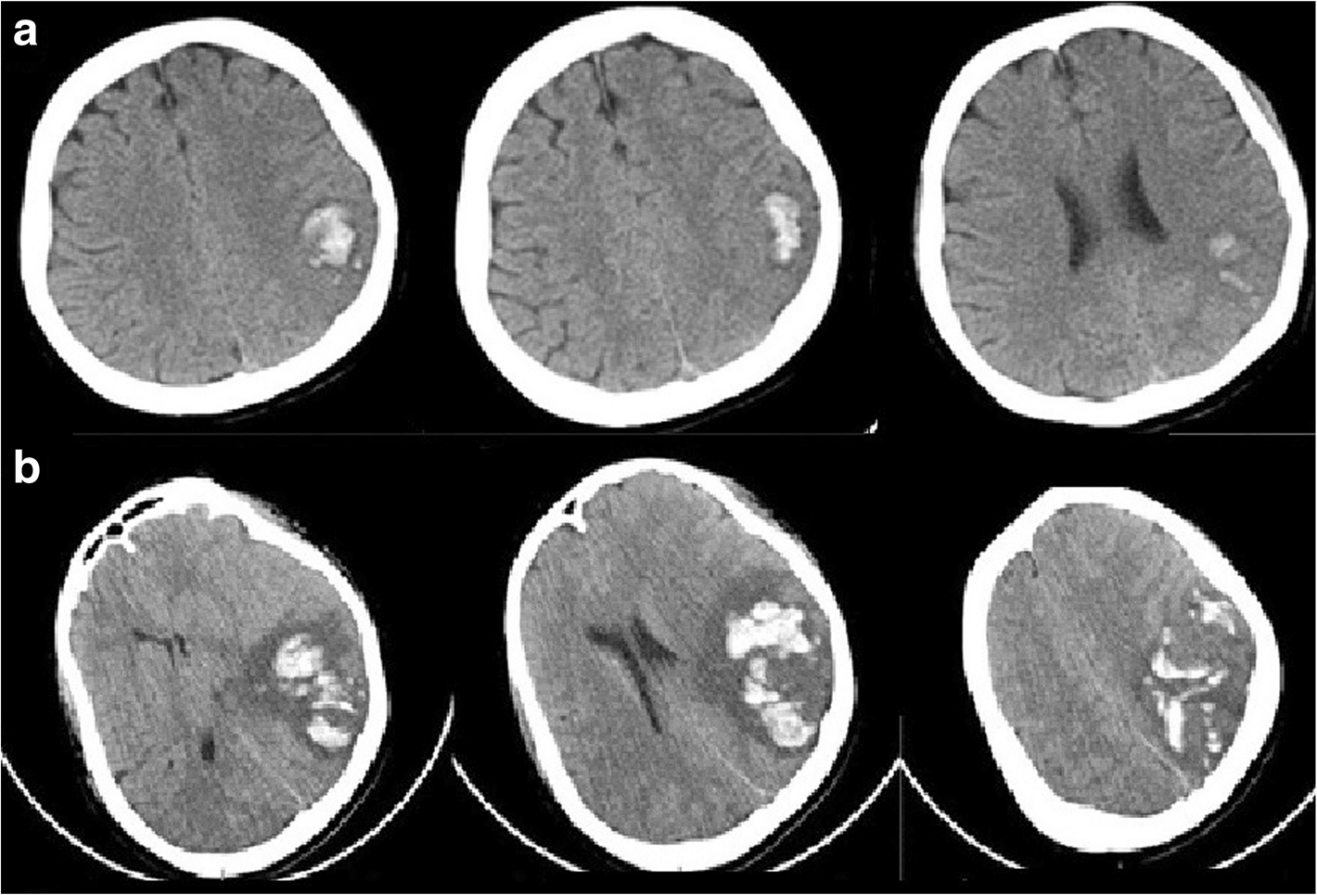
a Computed tomography images showing intracerebral hemorrhage in the left temporoparietal lobe, without brain midline shift before exacerbation. b Computed tomography images showing intracerebral hemorrhage expansion in the temporoparietal lobe and brain midline shift after exacerbation
